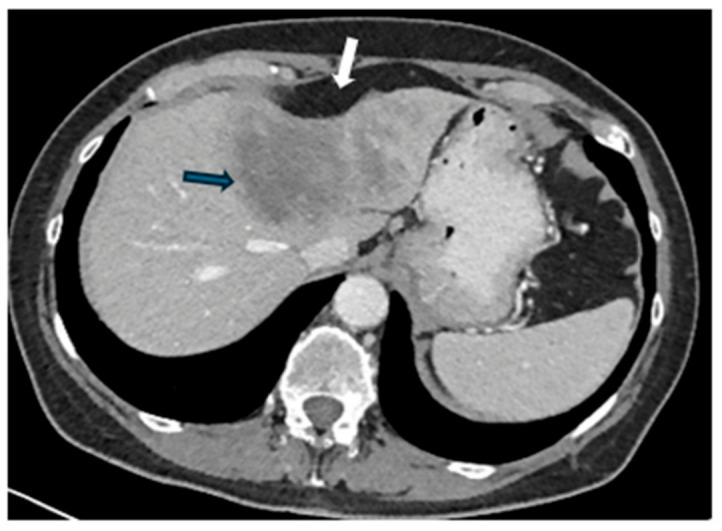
Axial contrast-enhanced computed tomography (CT) image demonstrating intrahepatic cholangiocarcinoma, seen as a large hypoenhancing mass (dark arrow) in the right hepatic lobe with irregular margins and associated capsular retraction (white arrow).
